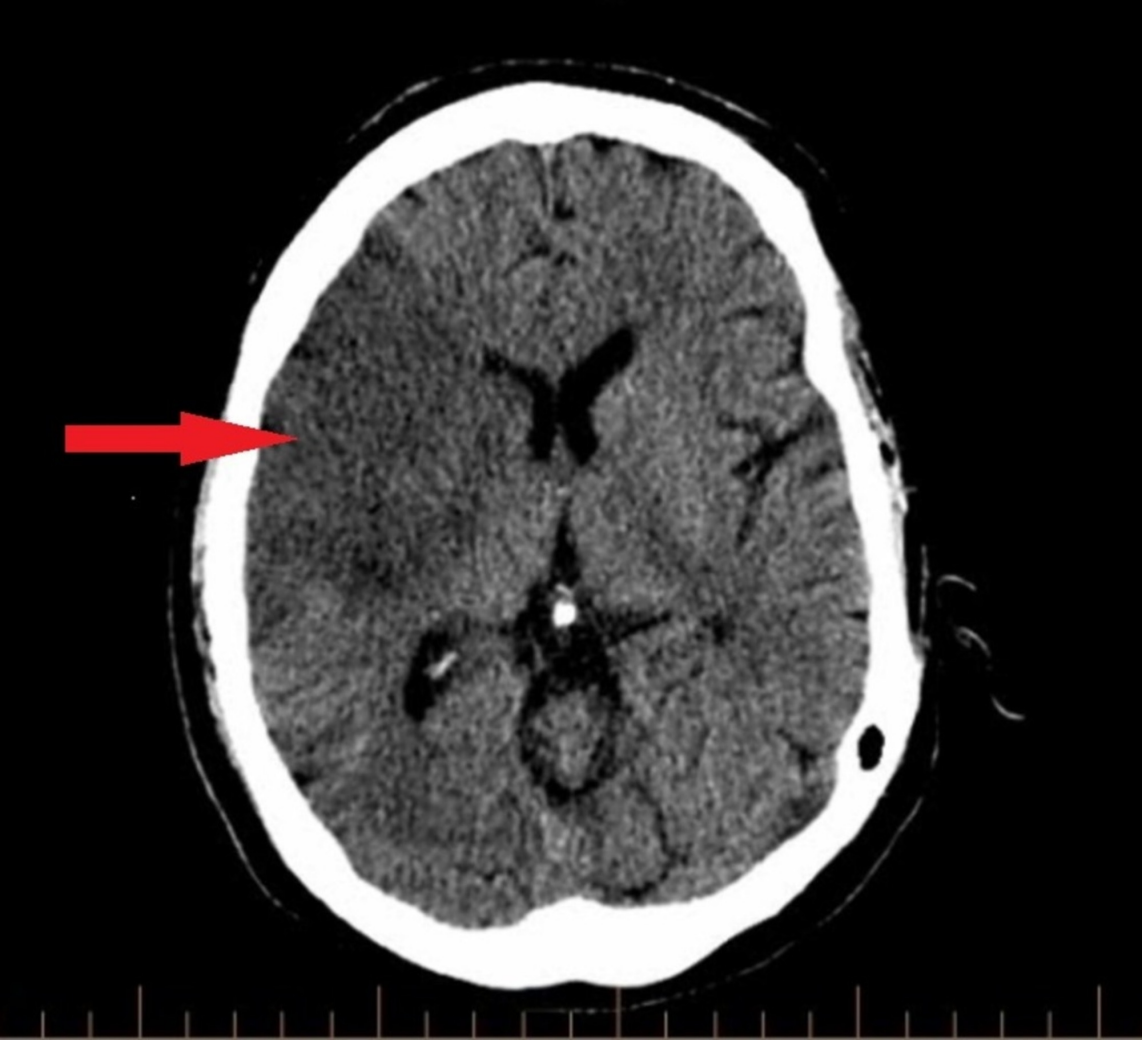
Noncontrast CT head showing a large right middle cerebral artery territory infarct involving the right frontal, right temporal, and right parietal lobes CT head demonstrating hypoattenuation of the right frontoparietotemporal cortex (red arrow) suggestive of ischemic infarct CT: computed tomography
